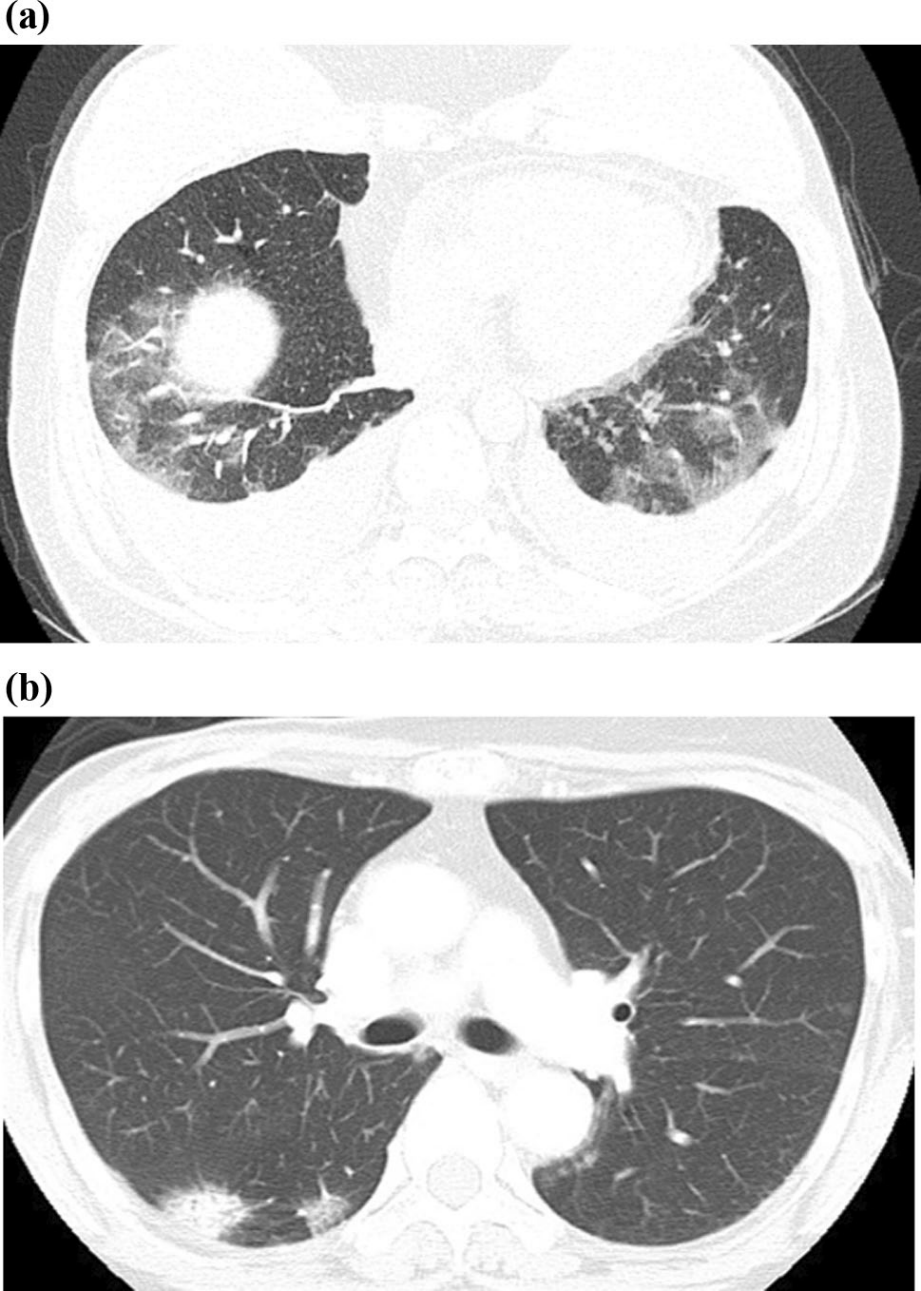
a, b Interstitial Lung Disease (ILD) in Two Patients Undergoing Systemic Therapy. a A woman in her 40s who underwent neoadjuvant chemotherapy (FEC followed by DTX) followed by endocrine therapy combined with a CDK4/6 inhibitor. She presented with follow-up CT findings suggesting ILD. b A different woman in her 40s with multiple metastases who was undergoing combined therapy with a CDK4/6 inhibitor. She complained of a cough and shortness of breath, and a CT scan revealed ILD. CT scans are essential for early detection of ILD, often identifying characteristic findings such as ground-glass opacities (a) or an organizing pneumonia pattern (b)
